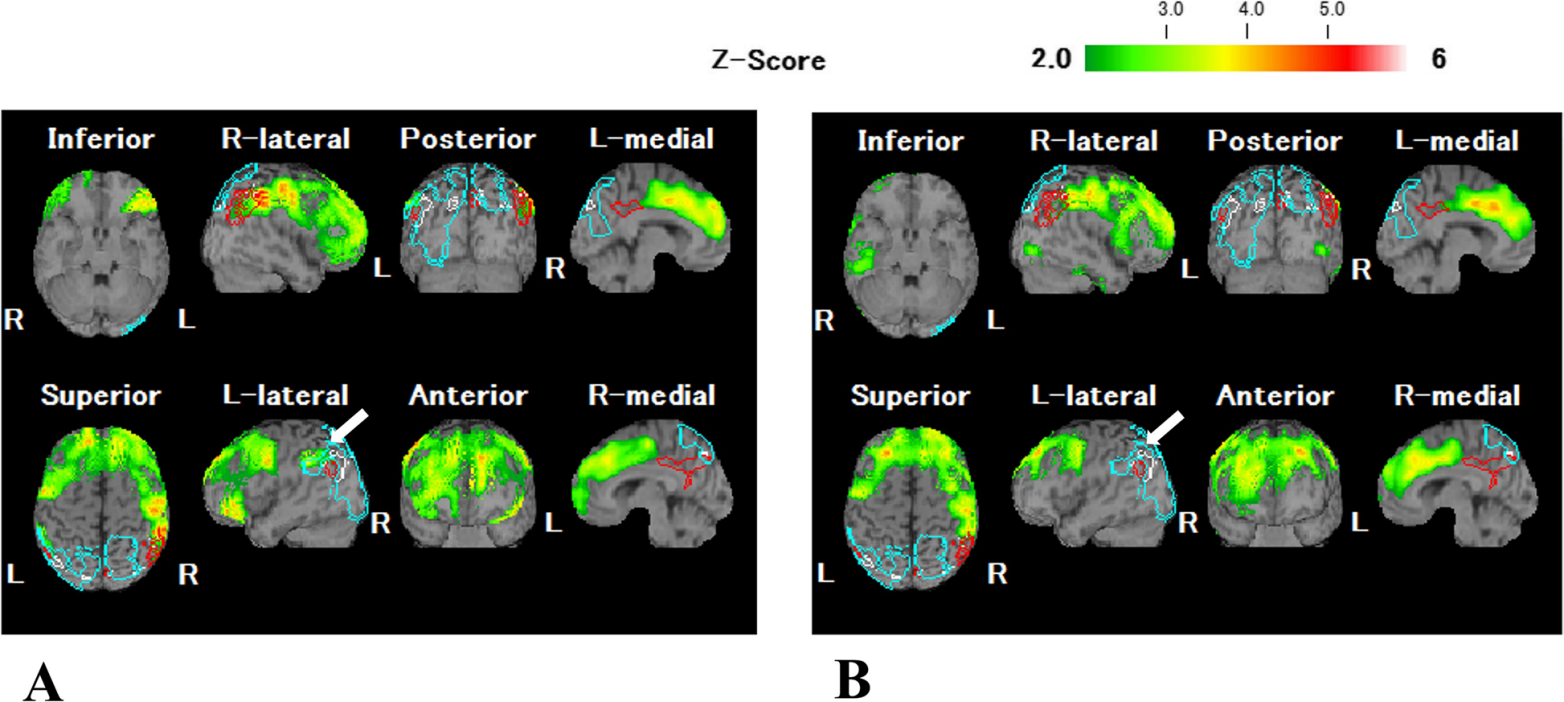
Serial single photon emission computed tomography (SEPCT) assessment. The easy Z-score analysis images for SPECT obtained 1 month (A) and 2 months (B) after the neurological symptom onset. Panel A shows decreased regional cerebral blood flow (rCBF) in the left supramarginal gyrus (arrow) in addition to the bilateral frontal cortices. Panel B shows improved rCBF in the left supramarginal gyrus (arrow)
